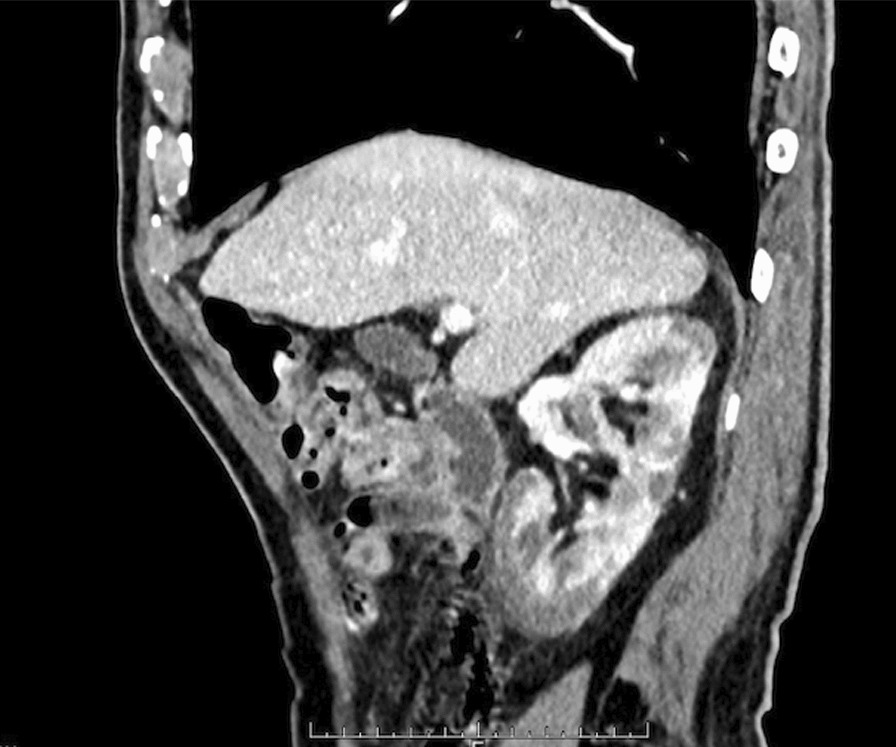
CT revealed that inflammation from the right kidney stimulated the diaphragm
